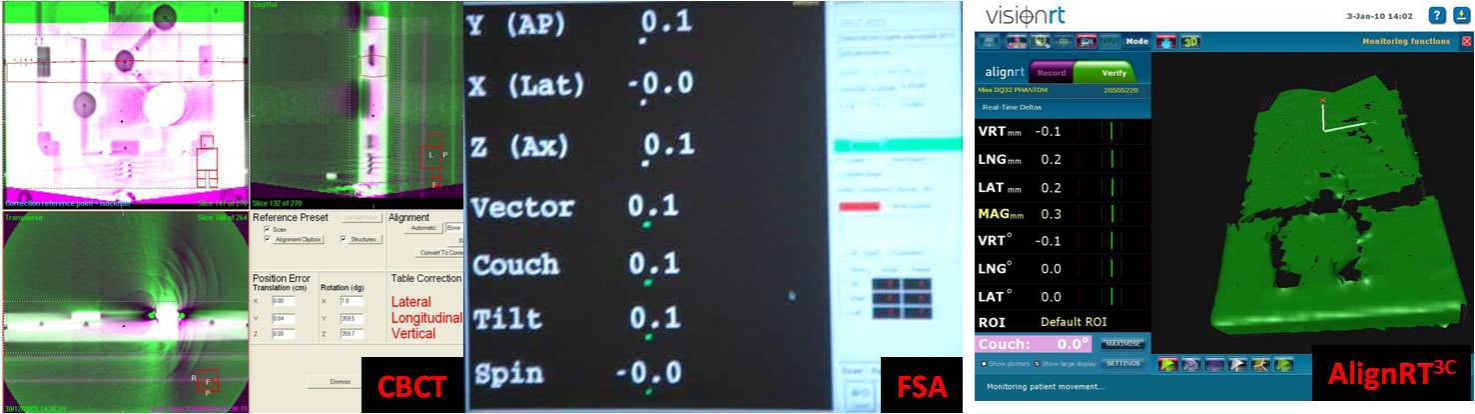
Examples of registration process for the three IGSPSs (CBCT, FSA, and ${\text{AlignRT}}^{3C}$).
